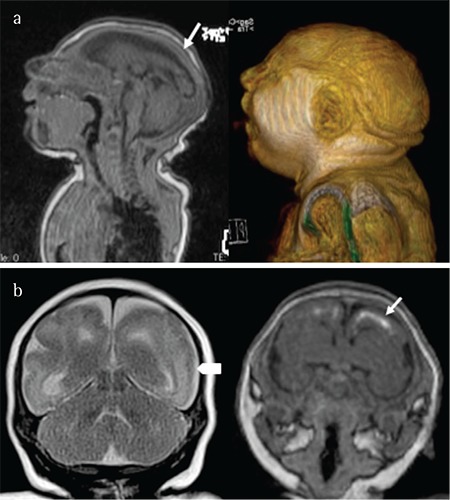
Postnatal T1-weighted magnetic resonance imaging in sagittal plane showing cortical atrophy (arrow) and three-dimensional reconstruction showing microcephaly (a), coronal T2- and T1-weight images showing smoothness of the brain (arrow head) and calcifications (arrow) (b)
